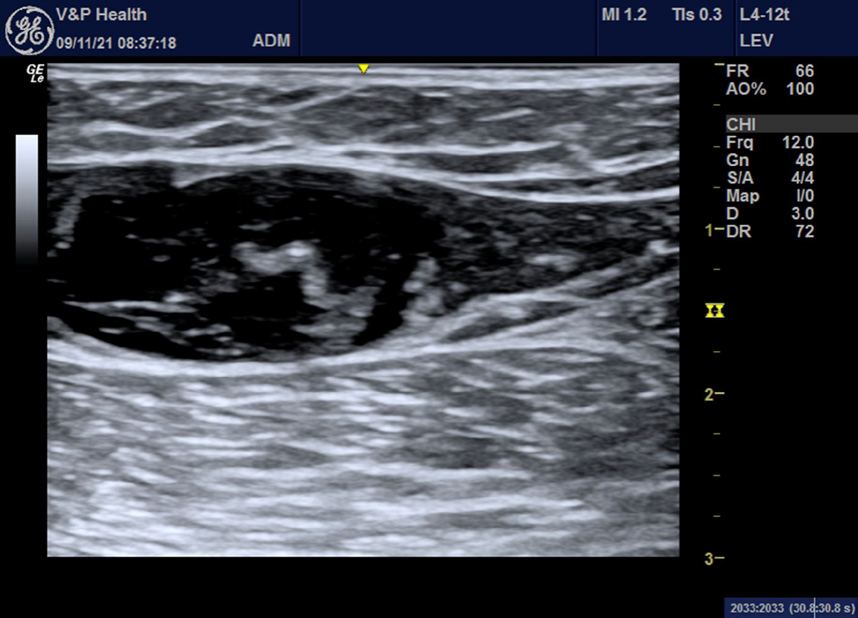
Color Doppler ultrasound image in B-mode showing the area of tumescence with the fiber central.9 - Thermal ablation of the GSV is performed using a 1,470 nm wavelength laser, following a proximal-to-distal thermal ablation sequence and adhering to the following energy parameters, depending on the characteristics of the vein being treated (Figure 4):
